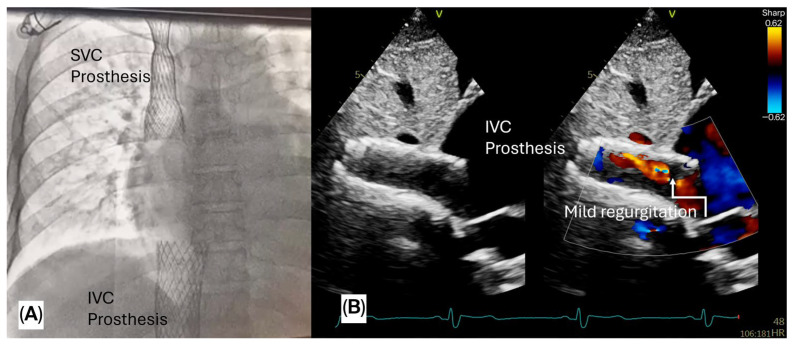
Heterotopic bicaval valve implantation using the TricValve system. (A) Fluoroscopy showing the final position of the prostheses in the superior (SVC) and inferior vena cavae (IVC). (B) Post-implant echocardiographic image from the subcostal view of the IVC prosthesis, with colour Doppler demonstrating mild residual regurgitation.
